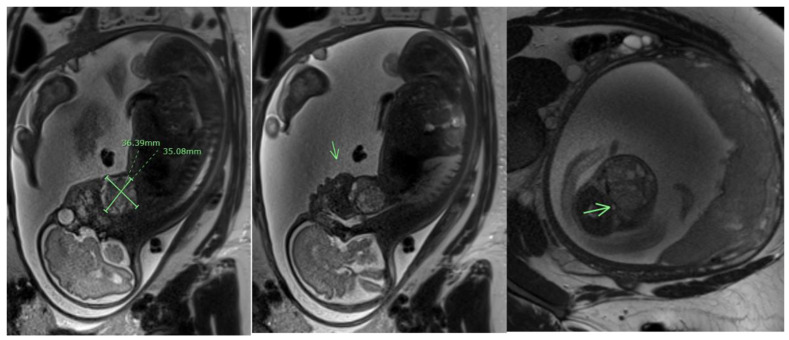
Fetal MRI of a cervical teratoma at 29 weeks and 1 day of gestation. Arrows point to the cervical teratoma.
